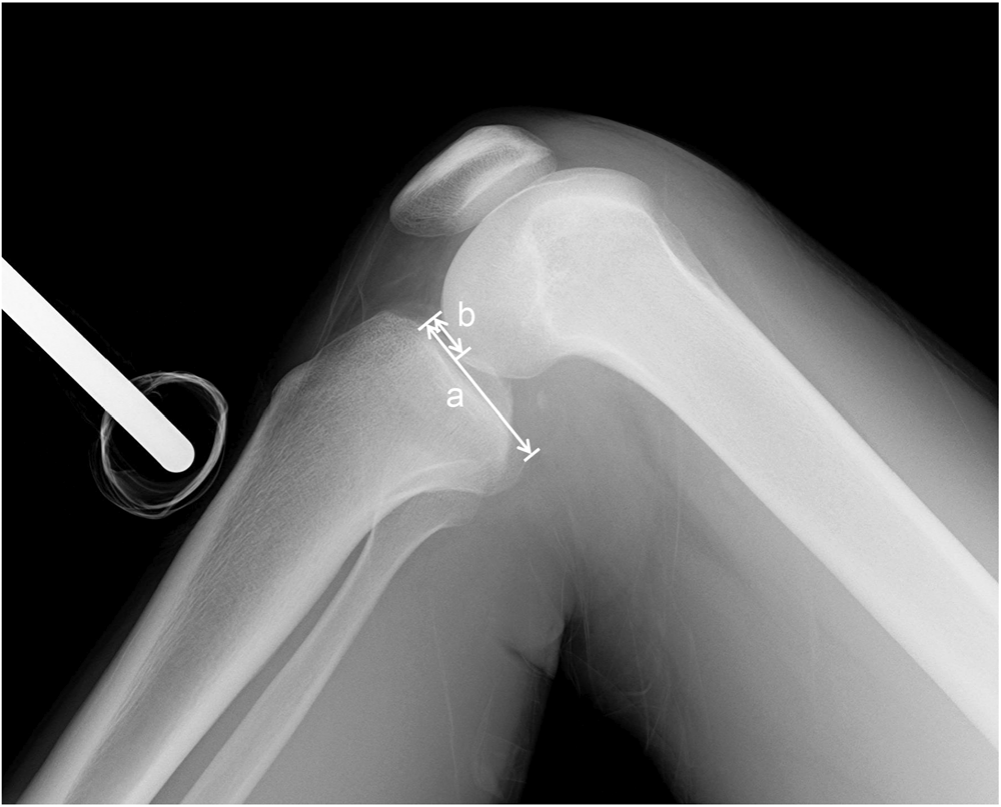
Evaluation of the femur‐tibia position on stress radiograph in 90° of knee flexion. A line was drawn along the tibial plateau, and b line parallel to the tibial plateau were drawn from the anterior of tibial plateau to the overlapped medial and lateral femoral posterior condyles. The ratio of the length from the anterior of tibial plateau to the midpoint of femoral posterior condyles and anterior to the posterior of tibial plateau was indicated as a percentage.
